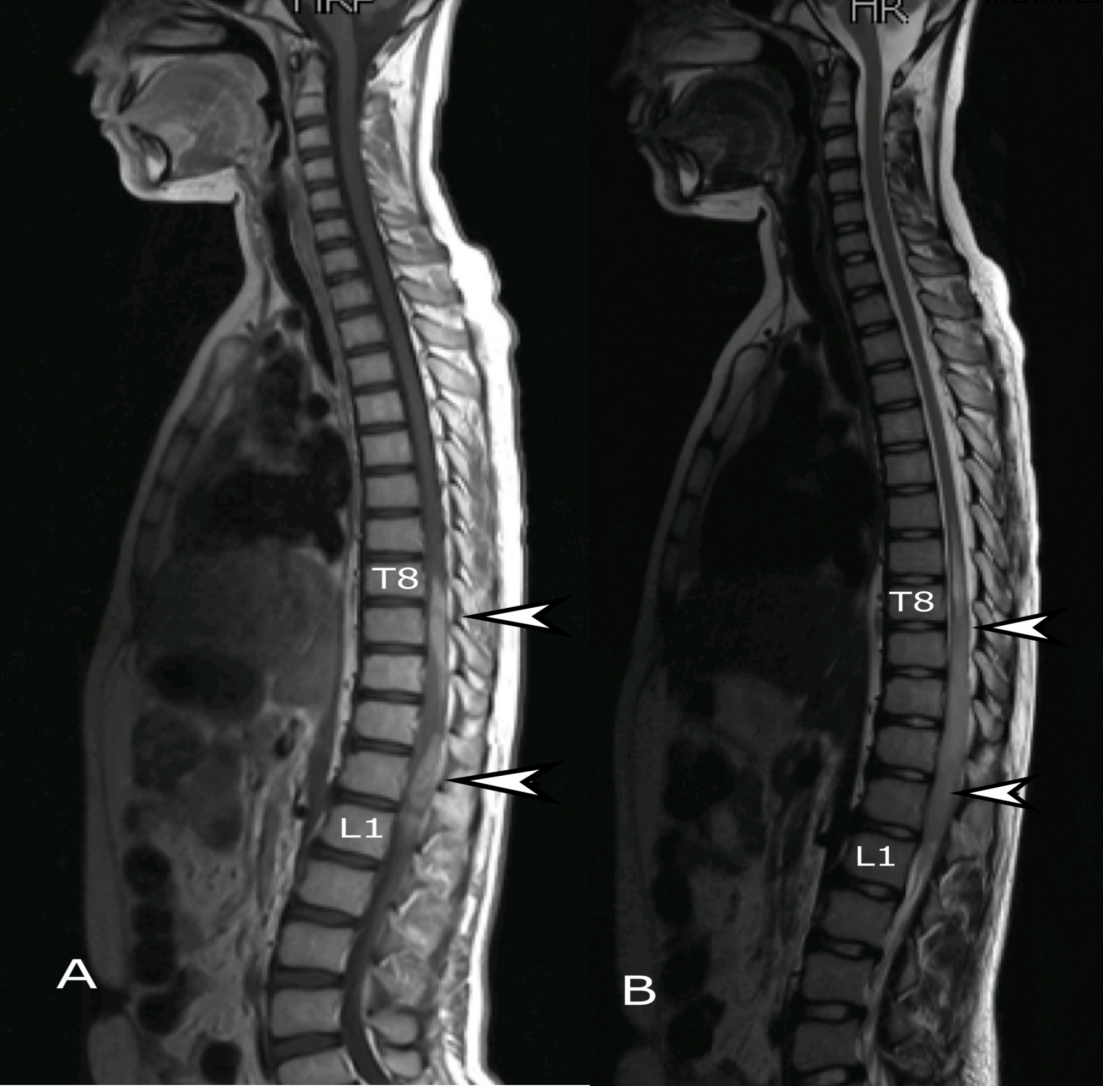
MRI whole spine, sagittal view. (A) T2 weighted and (B) T1 weighted post-contrast images showing intramedullary lesion (arrows) extending from T8 to L1. MRI: Magnetic resonance imaging.
